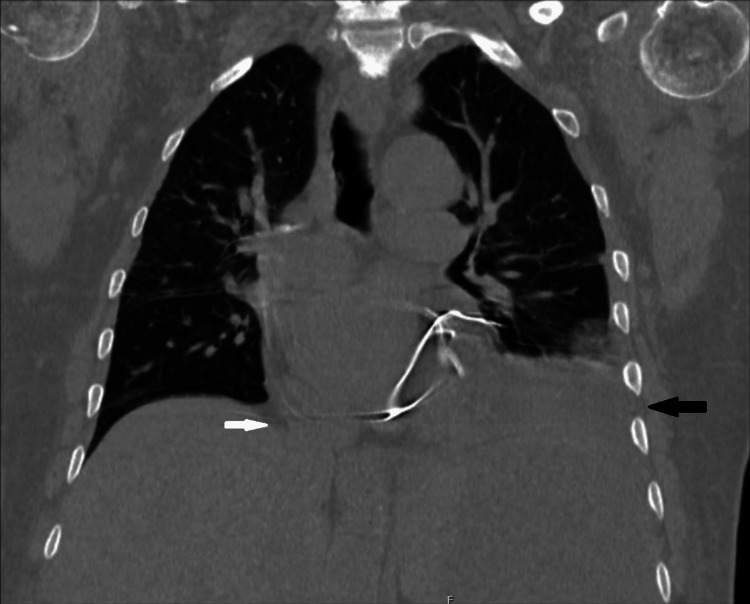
CT chest without contrast reveals pleural effusions and a large splenic mass CT chest without contrast reveals bilateral pleural effusions as well as a large splenic mass not seen on previous imaging at our facility.
